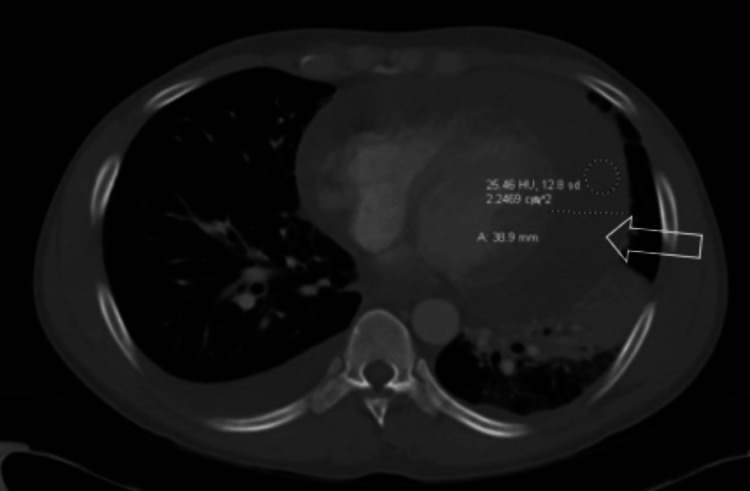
Large pericardial effusion visualized on CT
